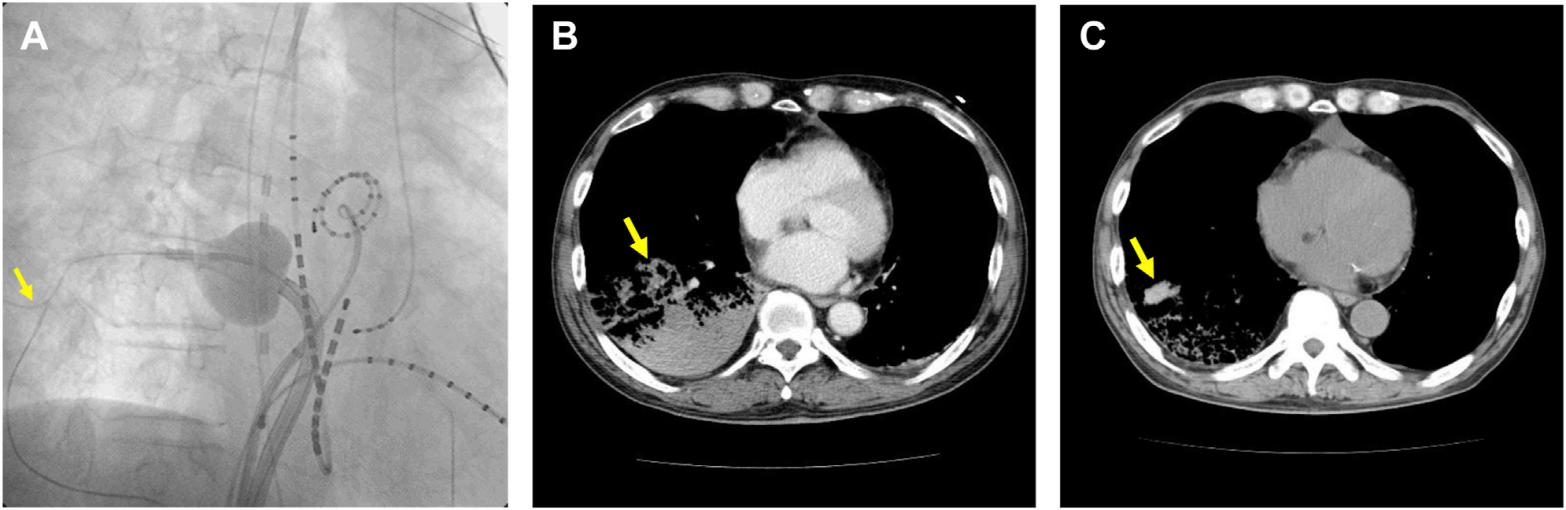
A: Fluoroscopic image during hot balloon ablation (HBA) of the right inferior pulmonary vein (RIPV). The yellow arrow indicates the guidewire advanced in the small branch of the RIPV in the fluoroscopic image. B, C: Computed tomography images immediately after (B) and 6 days after the HBA procedure (C). The yellow arrow in image B indicates the consolidation in the right lower lobe suggestive of a pulmonary hemorrhage. The yellow arrow in image C indicates that the pulmonary hemorrhage in the right lower lobe had improved.
